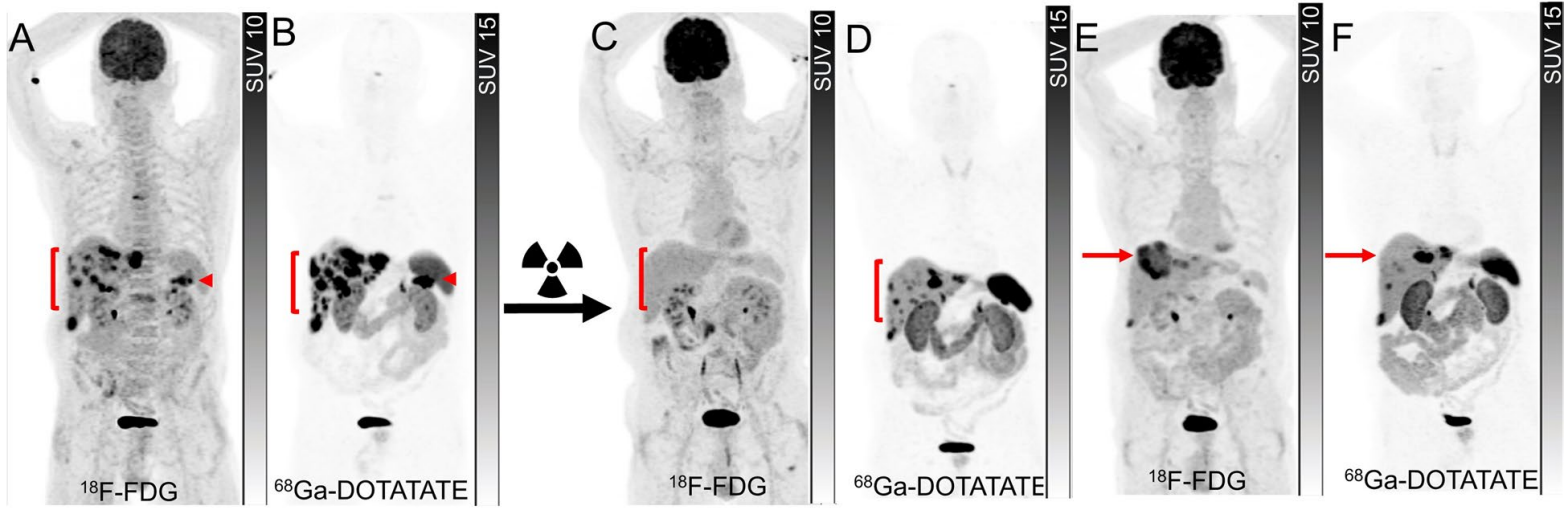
A patient with progressive metastatic pancreatic NET (G2, Ki67 10%) with glucagon hypersecretion with no prior treatments. ^18^F-FDG PET (A) and ^68^Ga-DOTATATE PET (B) showed a tracer avid lesion in the tail of the pancreas (arrowheads) and multiple hepatic metastases (brackets). As all lesions demonstrated intense ^68^Ga-DOTATATE uptake with no ^18^F-FDG-avid/non-^68^Ga-DOTATATE-avid disease, the disease was considered suitable for ^177^Lu-DOTATATE treatment. Restaging imaging 3 months after 4 cycles of ^177^Lu-DOTATATE showed complete metabolic response on ^18^F-FDG PET (C) and partial response on ^68^Ga-DOTATATE PET (D). Restaging ^18^F-FDG PET (E) and ^68^Ga-DOTATATE PET (F) 18 months later showed the development of an intensely ^18^F-FDG-avid/non-^68^Ga-DOTATATE-avid lesion in the dome of the liver (arrows), therefore the patient was deemed to be not suitable for retreatment with ^177^Lu-DOTATATE but considered for liver-directed therapy
